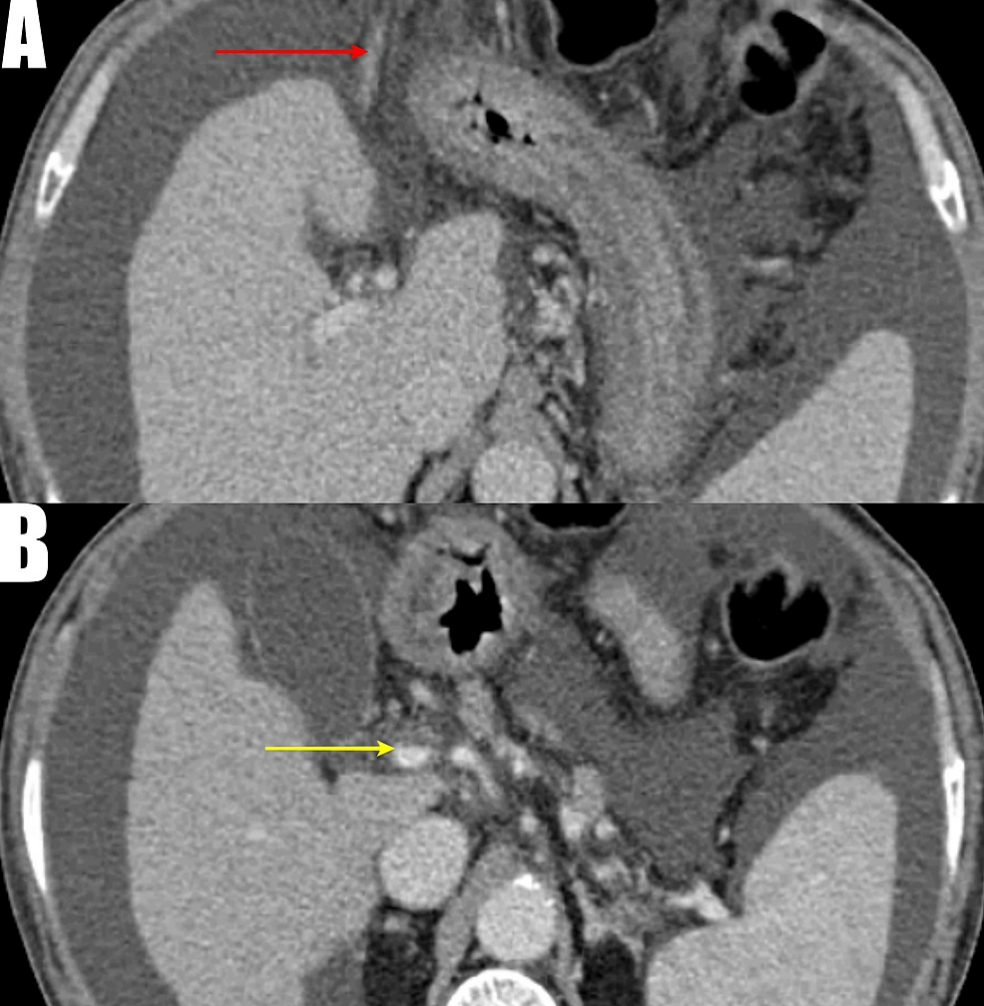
Computed tomography angiogram image A: Cirrhotic liver with massive ascites with a recanalized umbilical vein (red arrow); B: Thrombosis of the main portal vein marked by a yellow arrow
